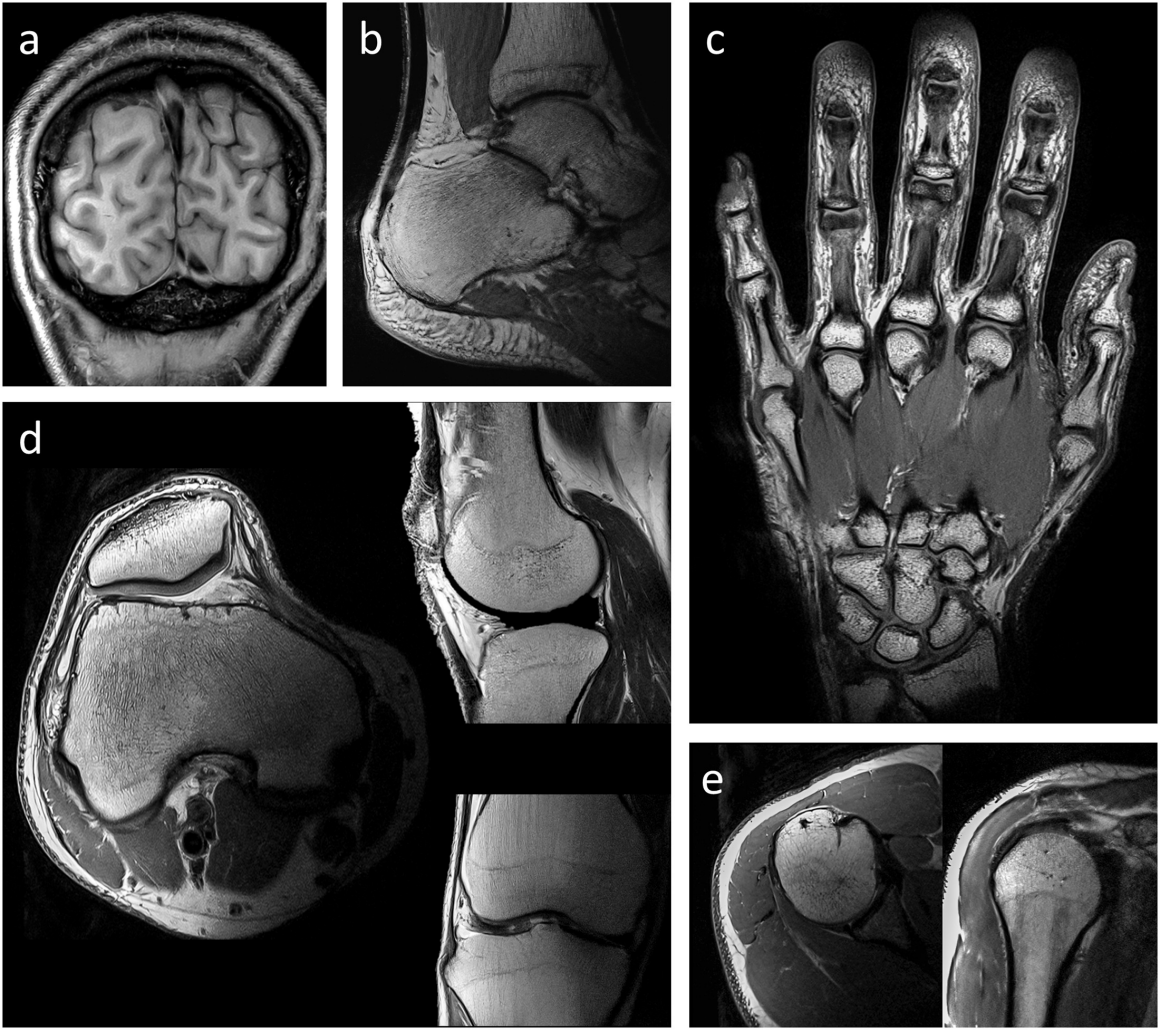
In vivo MR images. MR images showing image quality and versatility of the flexible coil array. a) occipital cortex, b) ankle, c) hand, d) knee in axial (left), sagittal (top right), and coronal (bottom right) view, e) shoulder in axial (left) and coronal (right) view. The corresponding imaging parameters are listed in Table 1.
